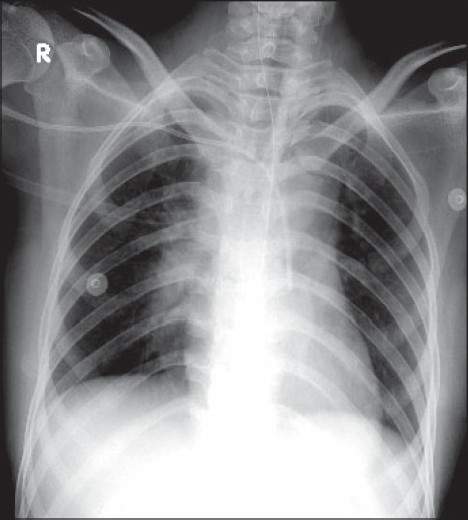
Radiograph of chest showing the tip of the catheter on the left side of the chest
